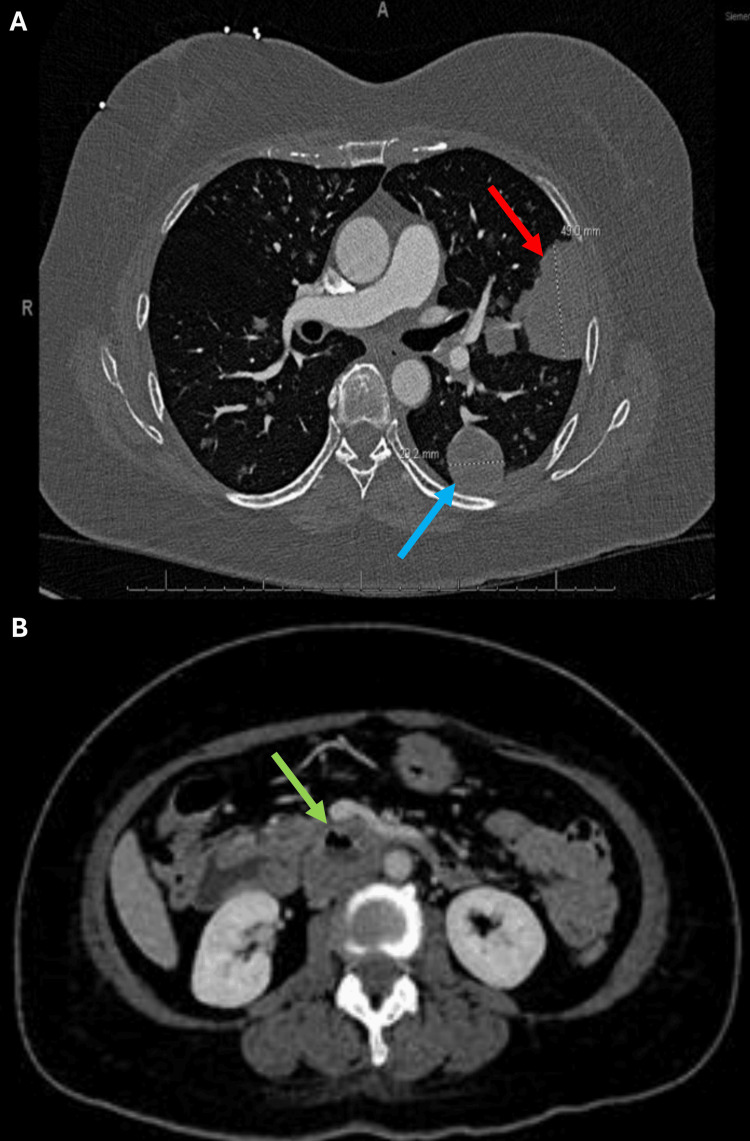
CT of the Chest, Abdomen, and Pelvis A: 4.9 cm left upper lobe peri-fissural mass (red arrow) and a 2.9 cm left lower lobe subpleural mass (blue arrow). B: A centrally necrotic 4.3 cm transverse duodenal mass (green arrow) narrowing the superior mesenteric vein and inferior vena cava.
